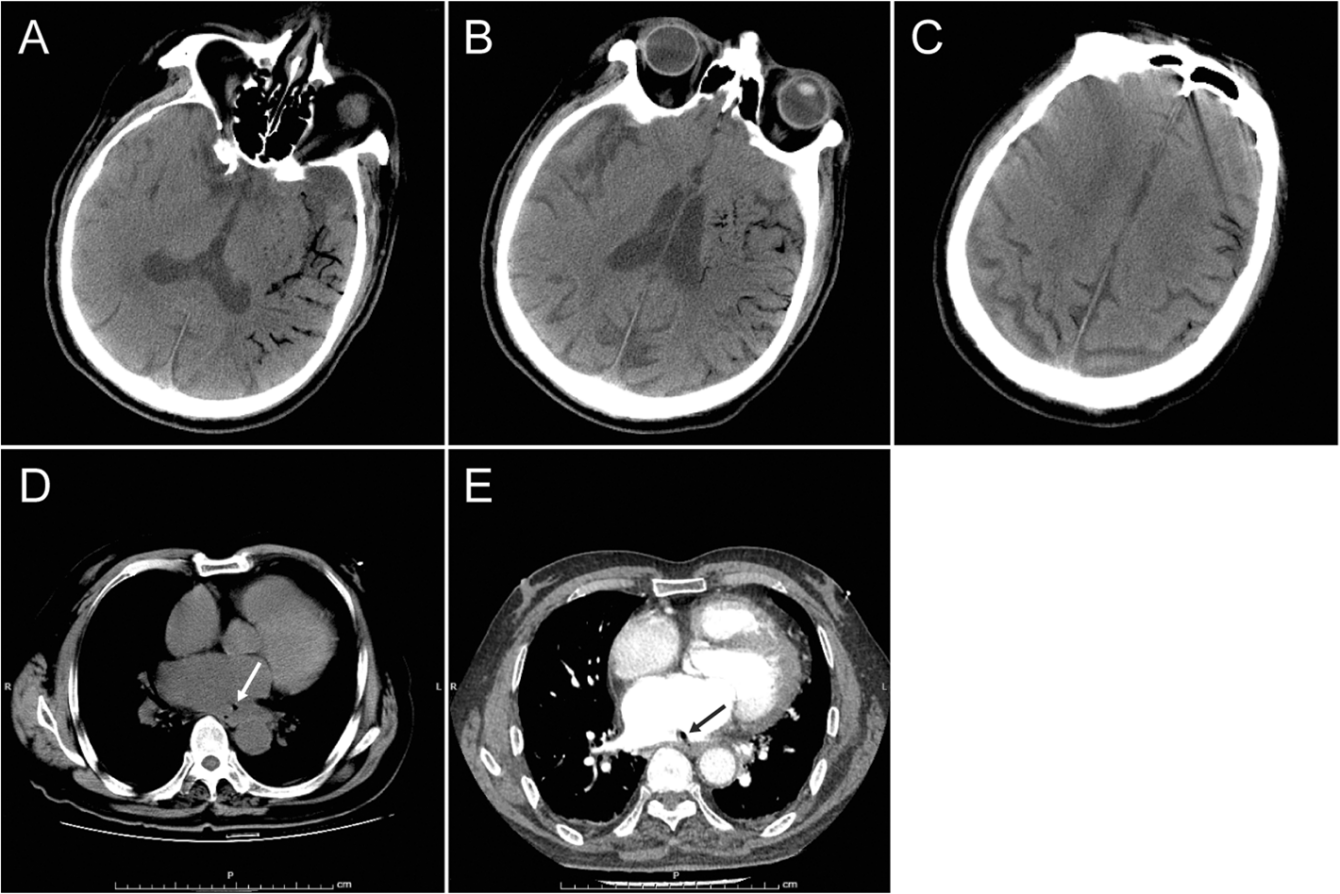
CT scanning. Brain CT shows massive air embolism in the left frontal, parietal, temporal, and occipital lobe (a, b, c), the left basal ganglion (b), as well as the right parietal lobe (c). Chest CT plain scan (d) and contrast-enhanced scan (e) show the enlargement of the left atrium, and a focus of air in the left atrium (arrows), which highly suggests an atrial-esophageal fistula
